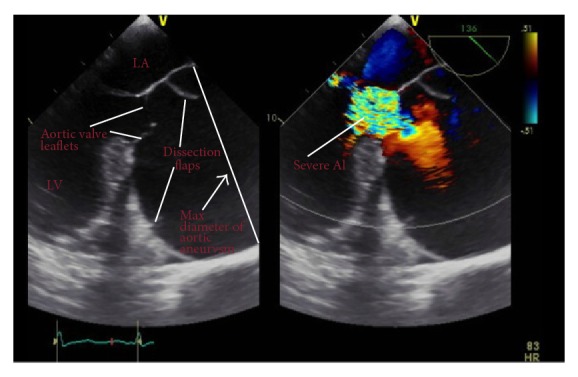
Transesophageal echocardiography in long axis aortic view showing giant aortic aneurysm (13 cm) and incidentally found dissection flaps in the ascending aorta (left). Also seen is severe aortic regurgitation (right). LA: left atrium, LV: left ventricle, and AI: aortic insufficiency.
